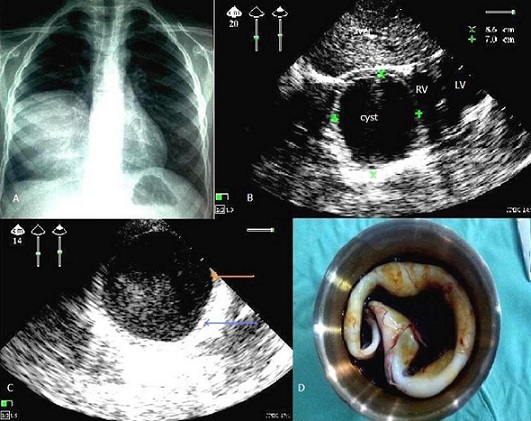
A) chest X-ray (face), showing a right basal opacity, although limited, fluid tonality; B) 2D ultrasound, subcostal through the liver window showing anechoic image with posterior reinforcement, well limited, right basal pulmonary, diameter 8.6/7 cm (RV: right ventricle, LV: left ventricle); C) 2D ultrasound, at right fifth intercostal space on the midclavicular line, showing an anechoic image with duplication of the wall: red arrow for the germinal membrane, blue arrow in relation to the reaction of the host, this is pathognomonic of hydatid cyst; D) operative part of the germinal membrane of the hydatid cyst
